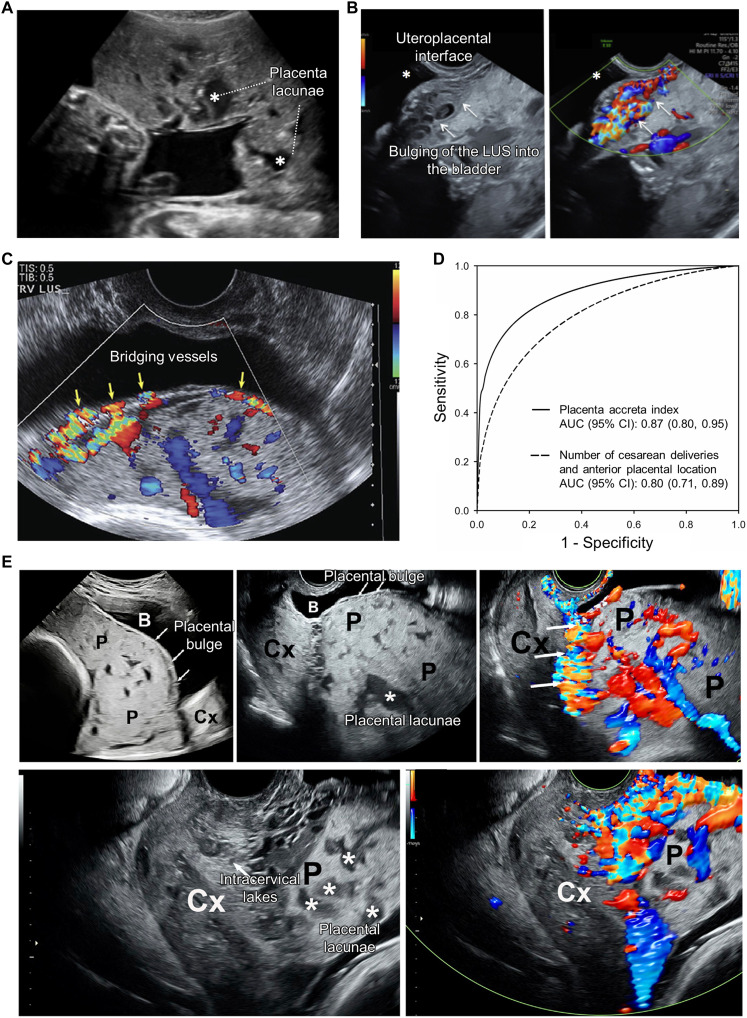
US imaging in clinical PAS studies. (A) B-mode image of placental lacunae. (B) Color Doppler image of the uteroplacental interface in PAS. LUS, lower uterine segment. (C) Color Doppler image of bridging vessels. (D) Placenta accreta index (PAI). 95% CI, 95% confidence interval. (E) Longitudinal B-mode and color Doppler images of the LUS and cervix (Cx) at 35 weeks of gestation in a patient with PAS. P, placenta previa. Figures are adapted from (*87*, *88*) (reused with permission) and (*90*) [CC BY (https://creativecommons.org/licenses/by/4.0/)].
